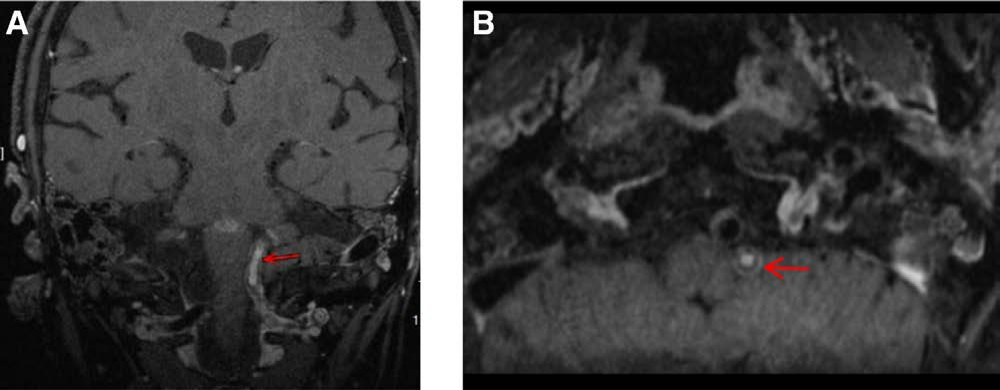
HR-MRI was performed to confirm LV4 thromboembolism (red arrow; (A) coronal view and (B) axial view).
